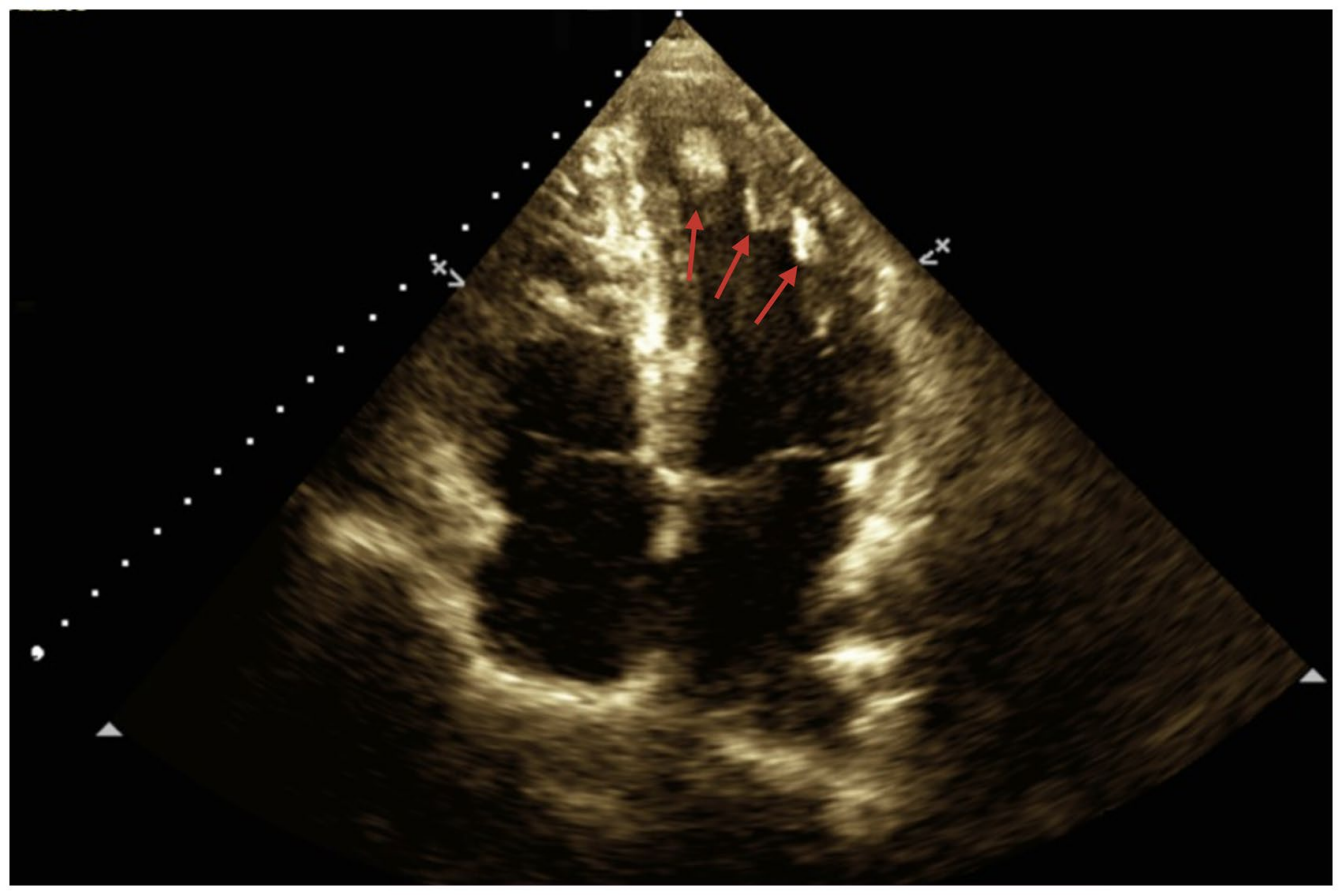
Two-dimensional echocardiography of the patient, showing an apical four-chamber view. The red arrows indicate several homogeneous masses with well-defined borders, retractile and without pedicles, affecting the entire interventricular septum and apical region. The largest mass, located closer to the interventricular septum, measures approximately 14 mm × 11 mm.
